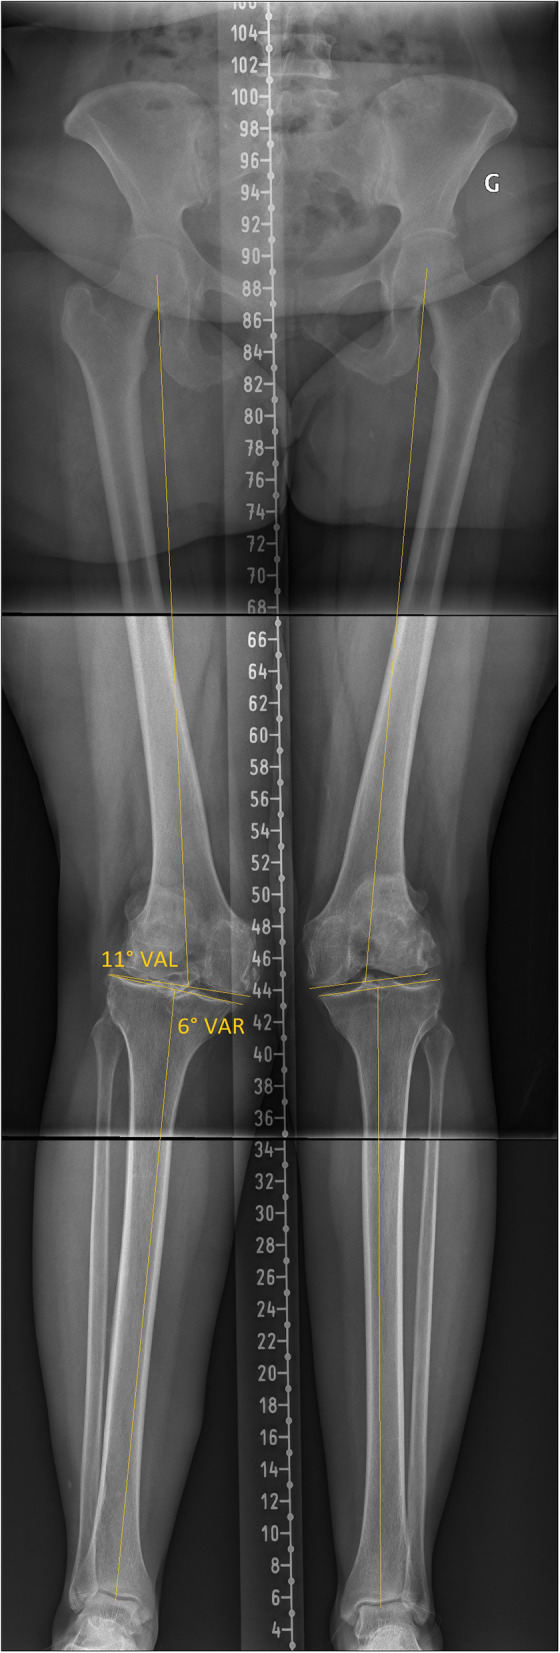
Lower limb full-length radiographs show bilateral valgus lower limbs with severe right knee OA. Her mDFA is 11° valgus and her mPTA is 6° varus. Applying unrestricted KA technique would reproduce her right lower limb alignment, reproducing extreme joint orientation (obliquity) and a lower limb arithmetic HKA (aHKA) in 5° of valgus. Such outlier anatomy might not be compatible with current TKA implant bearing and fixation methods.
